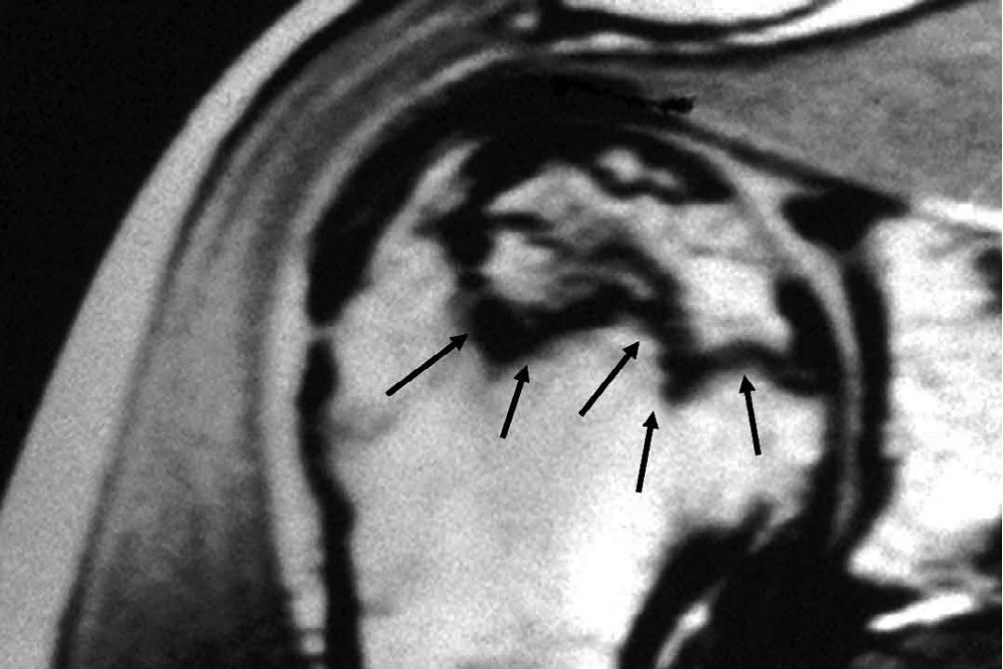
MRI appearance of early shoulder osteonecrosis: band‐like hypo‐intense (arrow) zones on T1‐weighted images demarcating the osteonecrosis.
